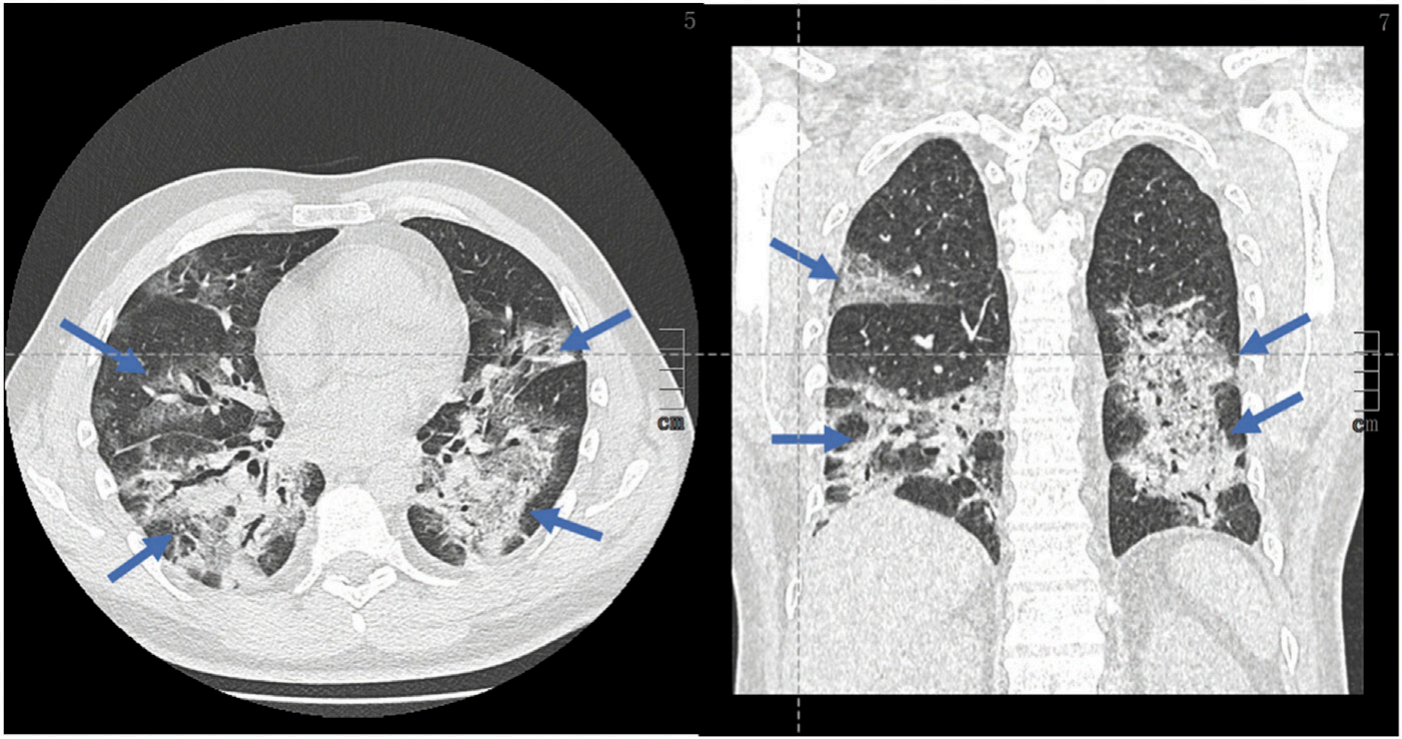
Chest computed tomography images of patients with COVID-19 pneumonia: shows extensive abnormalities and a proportion of pulmonary condensation (blue arrows) vs. higher Ground-glass, with possible progression to pulmonary fibrosis.
